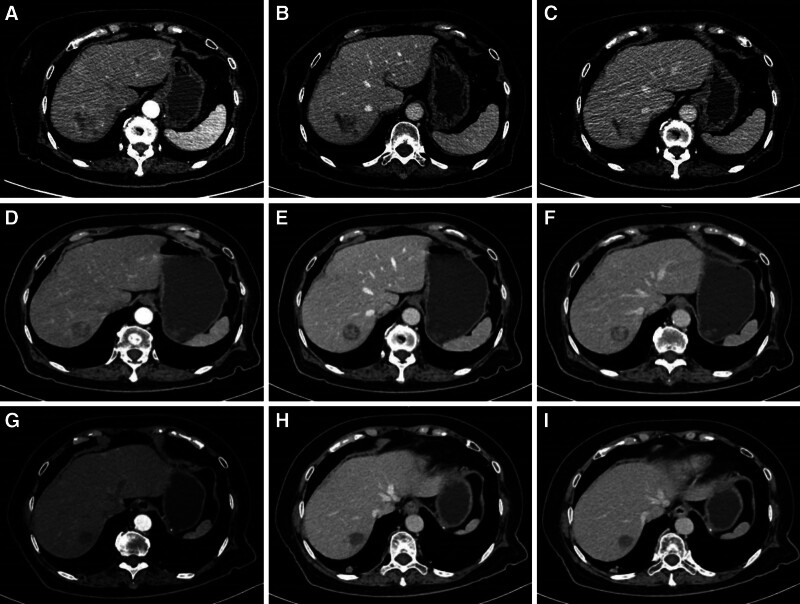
(A) The enhanced CT arterial phase after the first treatment; (B) The enhanced CT venous phase after the first treatment; (C) The enhanced CT delayed phase after the first treatment; (D) The enhanced CT arterial phase after the second treatment; (E) The enhanced CT venous phase after the second treatment; (F) The enhanced CT delayed phase after the second treatment; (G) The enhanced CT arterial phase after the third treatment; (H) The enhanced CT venous phase after the third treatment; and (I) The enhanced CT delayed phase after the third treatment. CT = computed tomography.
